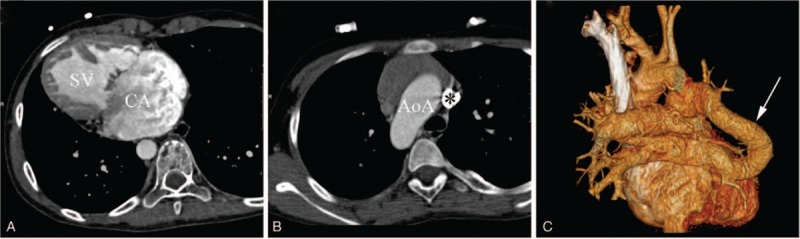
CA with indeterminate ventricle in a female aged 11 years. (A) Axial CT image reveals a CA with SV accompanied by dextrocardia. The morphological characteristics of SV cannot be identified. (B) Axial CT image shows a right-sided AoA and a persistent left superior vena cava (∗). (C) VR image shows a drainage of left pulmonary veins and partial right pulmonary veins into the right superior vena cava. AoA = aortic arch, CA = common atrium, SV = single ventricle, VR = volume reconstruction.
